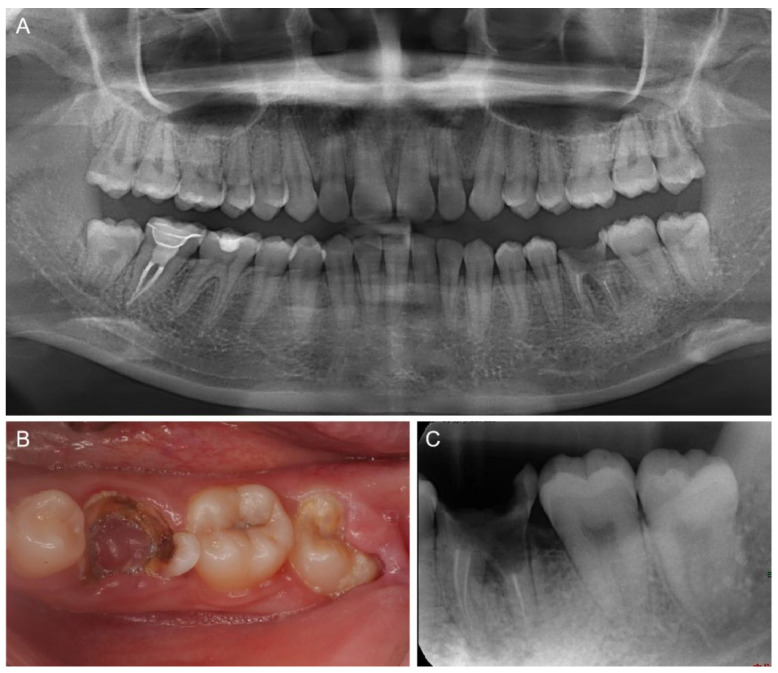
Preoperative examination. (A) A panoramic radiograph and (B) a clinical photograph showing tooth #36 with extensive caries and pulp polyp, and tooth #38 erupted partly. (C) Preoperative periapical radiograph showing tooth #36 with destruction on the floor of the pulp chamber and tooth #38 with completely formed roots.
